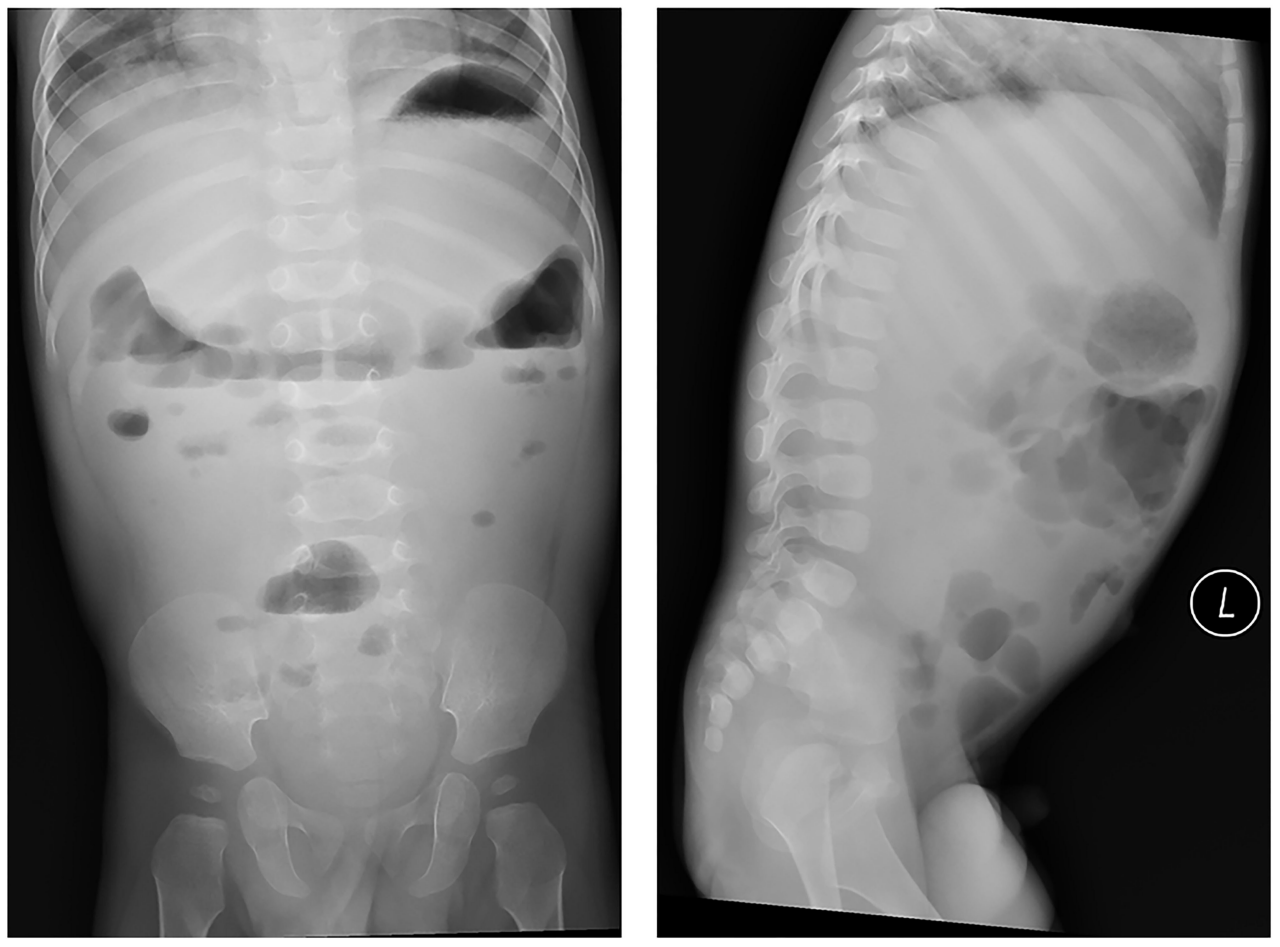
Abdominal radiographs suggested intestinal motility changes without any signs of intestinal obstruction.
